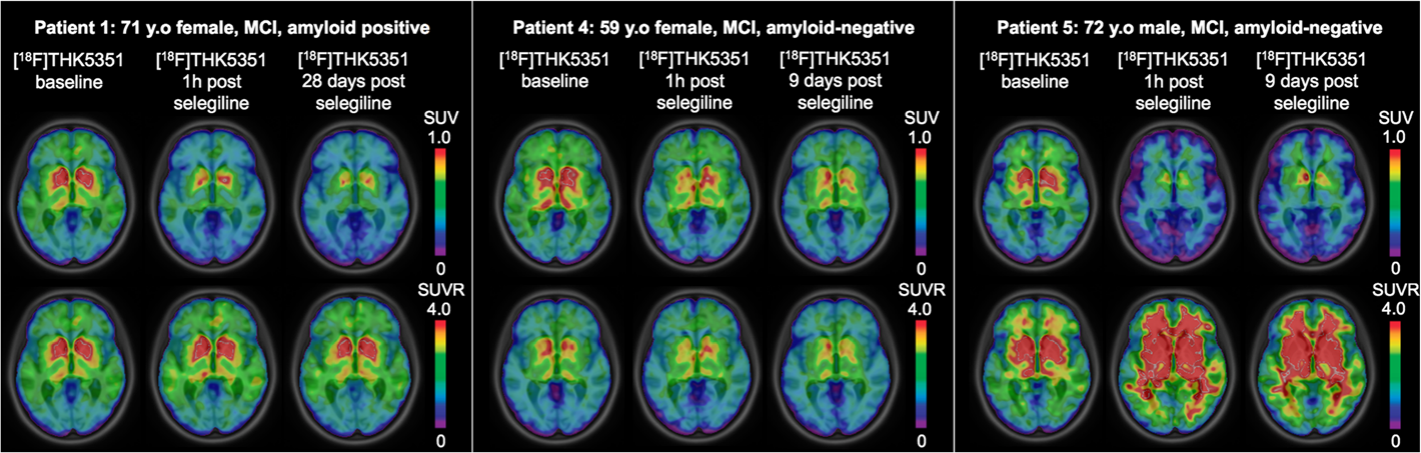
The reduction of ^18^F-THK5351 standardized uptake value remains in the second follow-up. Standardized uptake value (*SUV*) and SUV ratio (*SUVR*) map overlaid on a structural MRI scan showing the baseline, 1 h and 9–28 days post-selegiline SUV and SUVR from 50 to 70 min after ^18^F-THK5351 injection in three patients. *MCI* mild cognitive impairment, *y.o* years old
